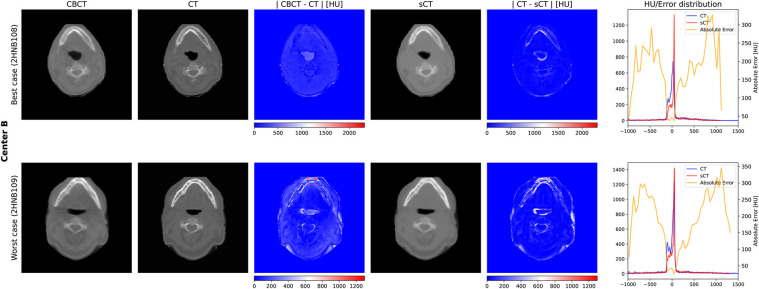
Qualitative results for the best and worst sCT cases from Center B, which showed lower image similarity metrics among the training centers. Widespread registration artifacts between CBCT and CT scans contributed to the visible errors. This was especially evident in the oral cavity of the 2HNB109 patient, and are reflected in both |CBCT−CT| figure and error map.
